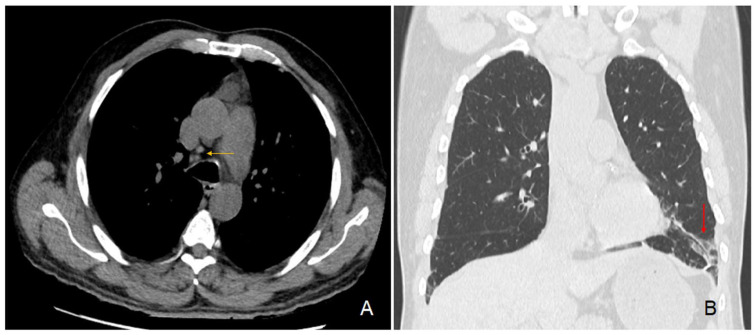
Chest CT scan images. Legend: transversal section (A) showing hilar lymphadenopathies (yellow arrow); coronal section (B) showing fibrous reticulations with bronchiolectasis of the pulmonary base (red arrow).
